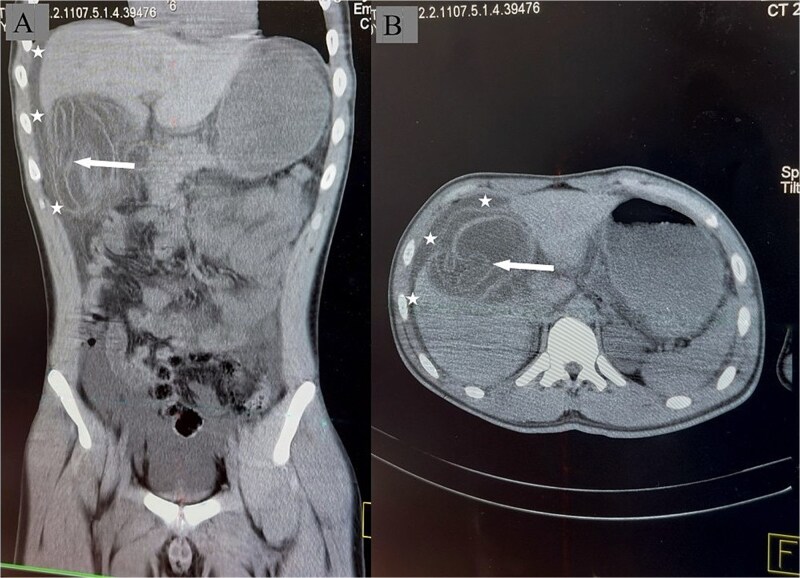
Non-contrast CT of the abdomen demonstrating a ruptured hepatic hydatid cyst. (A) coronal view showing the craniocaudal extent of the complex cystic lesion with undulating membrane (arrow). Free fluid is seen tracking from the subhepatic region to the subphrenic space (asterisk). (B) Axial view demonstrating the cystic lesion in the liver with the undulating laminated membrane the ‘water-lily sign’ being visible within the cyst cavity (arrow). Free fluid is present at the perihepatic regions (asterisk). No intervening liver parenchyma is seen between the undulating membrane of the cystic lesion and the peritoneal cavity, highly suggesting rupture to the peritoneum.
